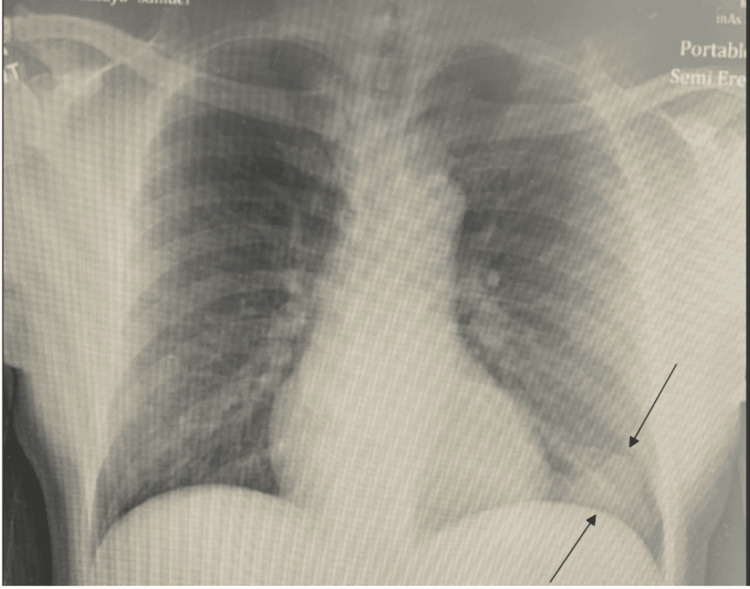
CXR showing pleural-based density measuring 4.1 cm (black arrows) CXR: chest X-ray
